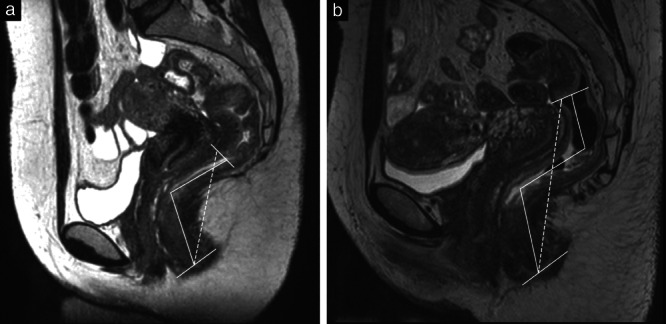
Magnetic resonance images (MRI) in two patients with rectosigmoid endometriosis, showing measurements of lesion‐to‐anal‐verge distance using MRI_Direct_ (
